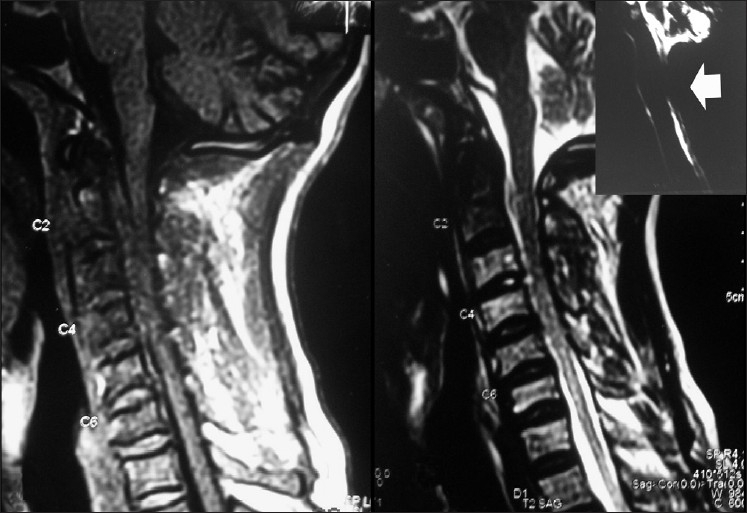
Magnetic resonance imaging of the cervical spine T1W and T2W sagittal images showing C3-5 cord compression
